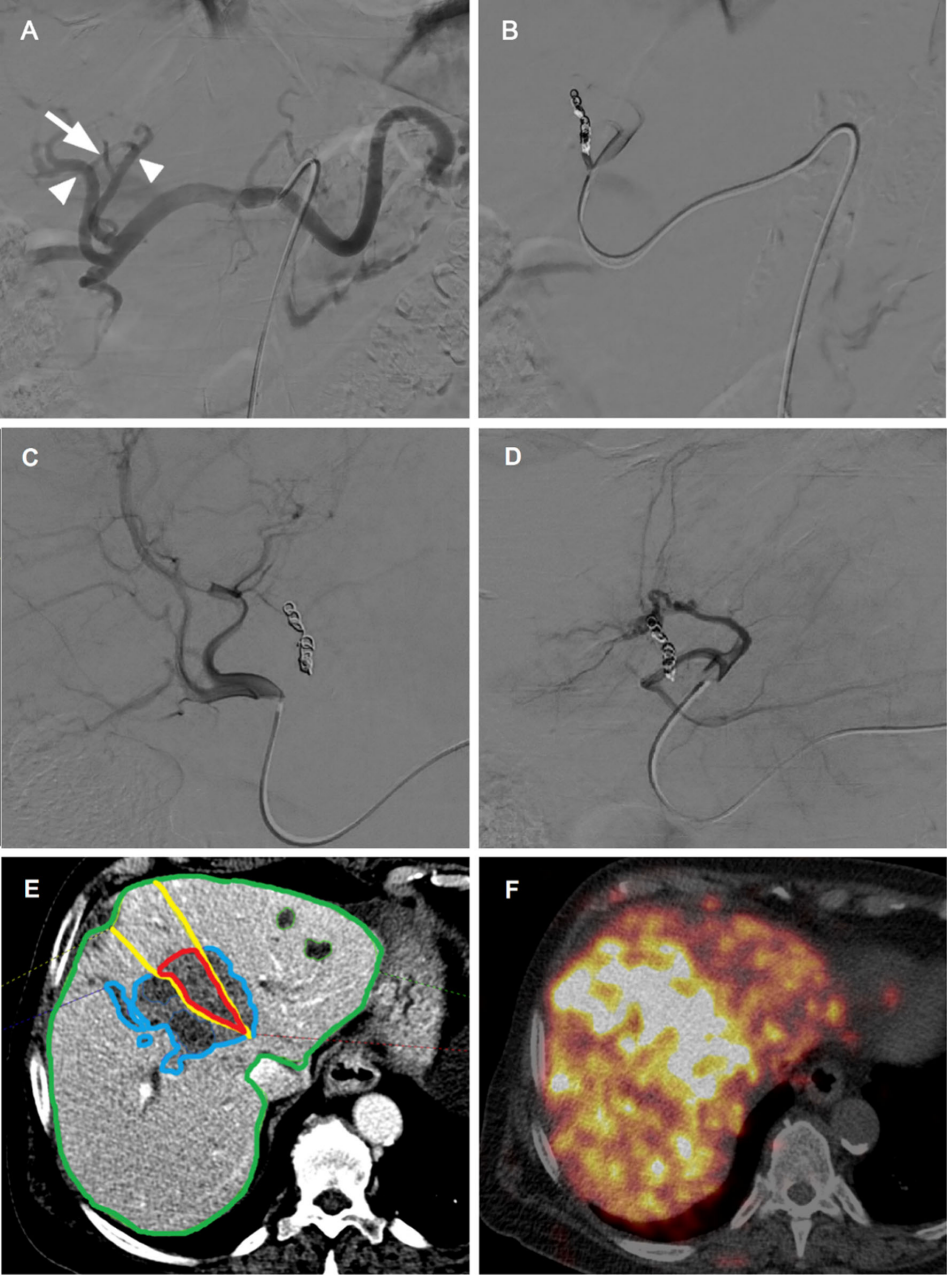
Example of successful redistribution in a patient with cholangiocarcinoma. A DSA showing the liver vasculature including the left hepatic artery origin of the segment IV branch (white arrow), as well as the future microsphere injection positions (white arrowheads). B Coil embolization of the segment IV branch. C Injection position in the RHA post-coil embolization. D Injection position in the LHA post-coil embolization. D Volumes of interest drawn using Simplicit^90^Y™ software, the dependent segment (IV) was drawn based on Couinaud’s classification of segmental anatomy. E^90^Y-PET/CT after treatment demonstrates a high concentration of microspheres throughout the liver, especially in segment IV. *p* = .162
